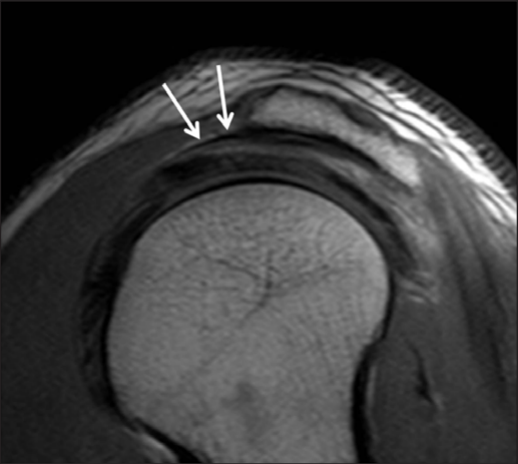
Subacromial pseudospur. Sagittal oblique PD-weighted MR image demonstrates the normal coracoacromial ligament at its acromial attachment that may mimic an osteophyte (arrows).
